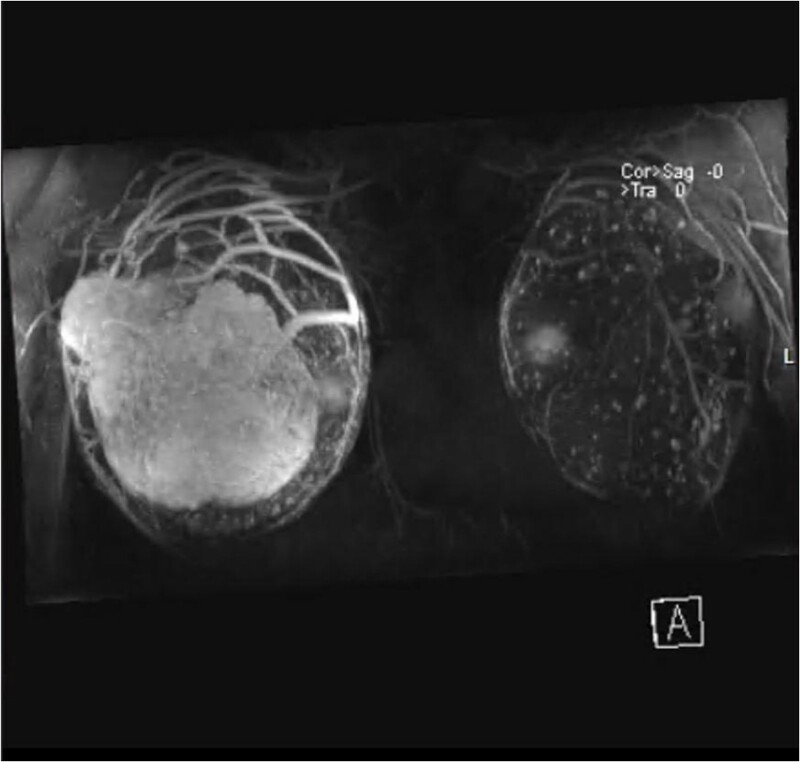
MRI with contrast of right breast and left breast.
